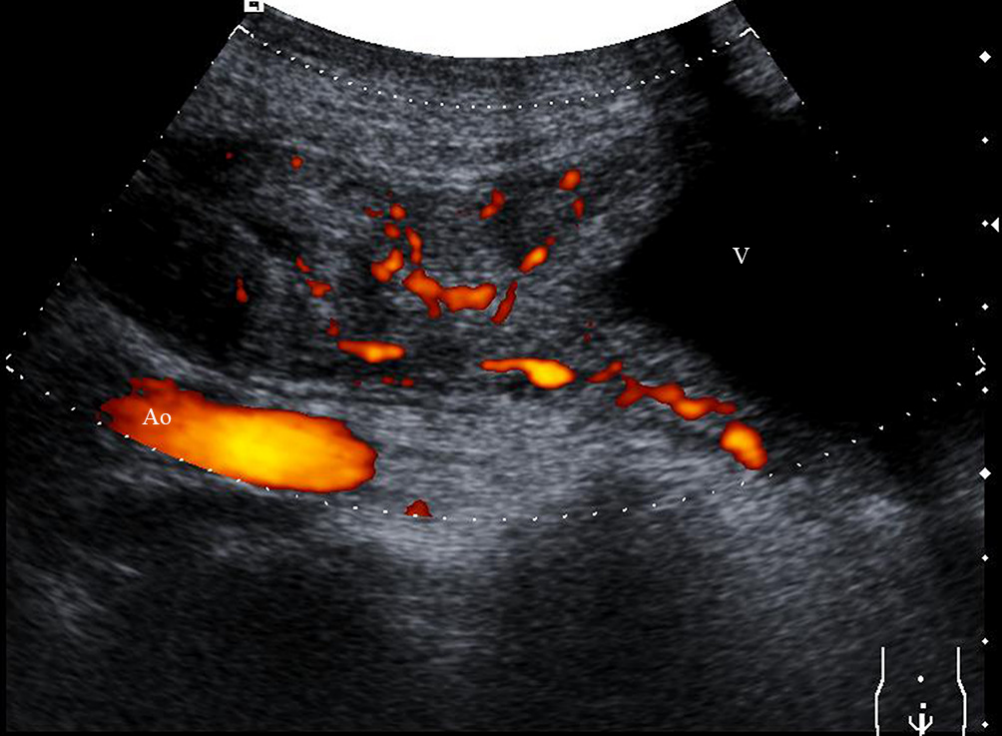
Longitudinal scan colour power Doppler showing hypoechoic lesion anterior to the aorta (Ao) with increased vascularity; the triangular aspect of the vesical dome (V) is noteworthy.
